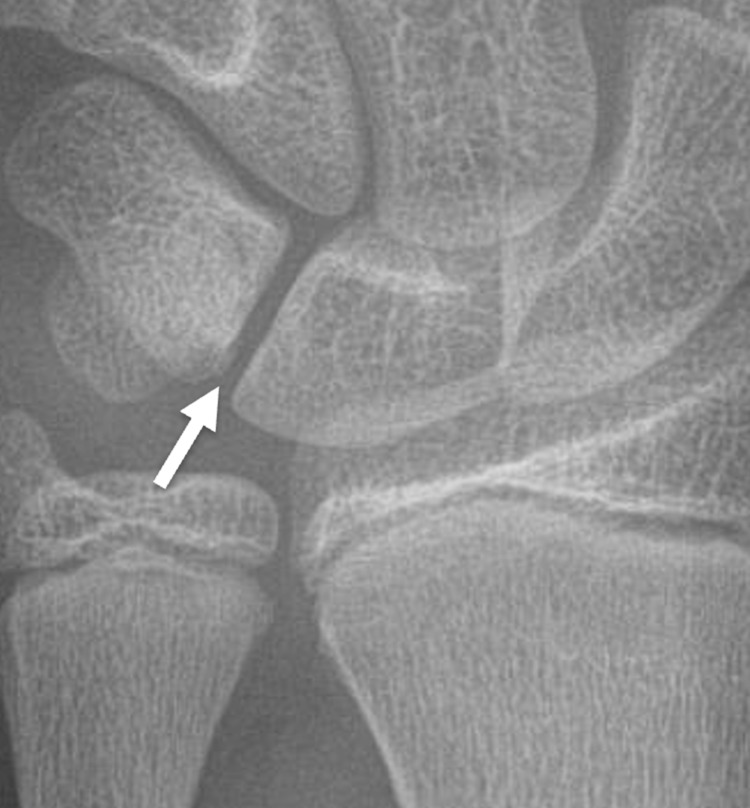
Magnified image of the same child's initial frontal wrist radiograph There are very early radiographic findings of a lunotriquetral coalition with close apposition of the proximal aspects of the lunate and triquetrum with a ‘parallel cortex’ appearance (arrow).
